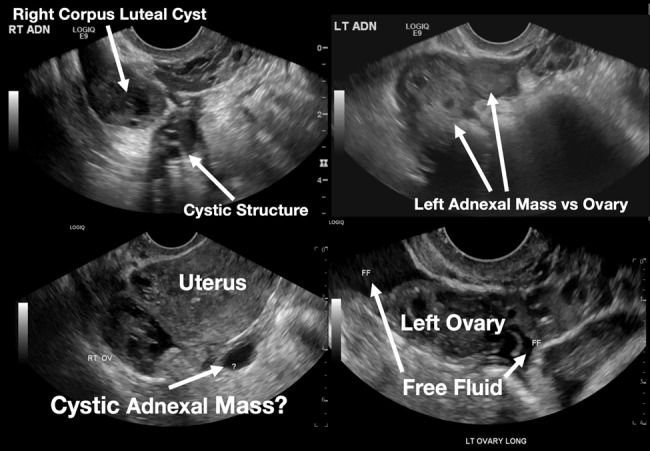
This image shows both of the patient’s ultrasound findings as described above for ease of comparison. The upper panel is from the initial encounter and the lower panel is from the patient’s encounter 11 days later.
